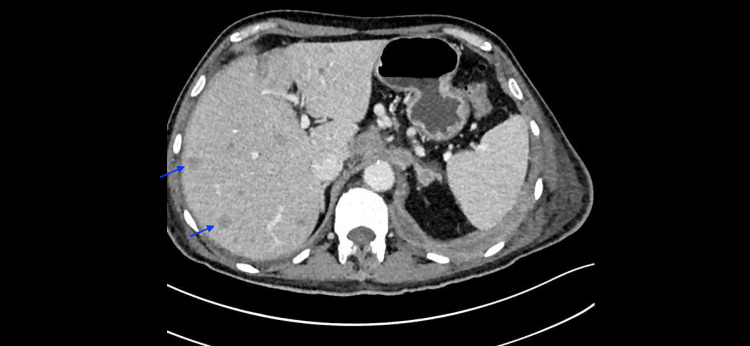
Abdominal CT scan image The image shows liver metastases (blue arrows). CT: Computed tomography.
